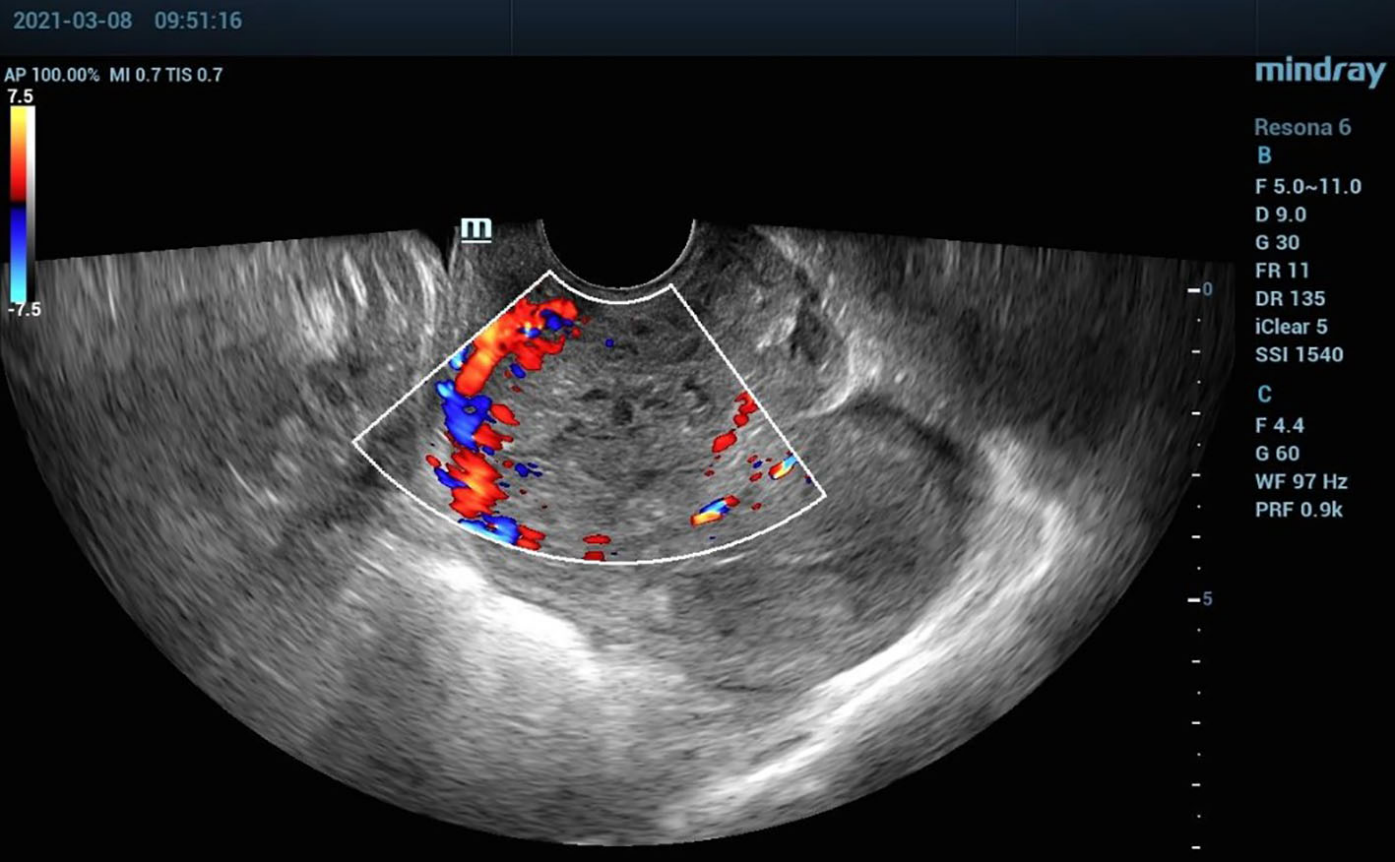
Ultrasound color Doppler image of the cervix, showing a highly vascularized zone.
